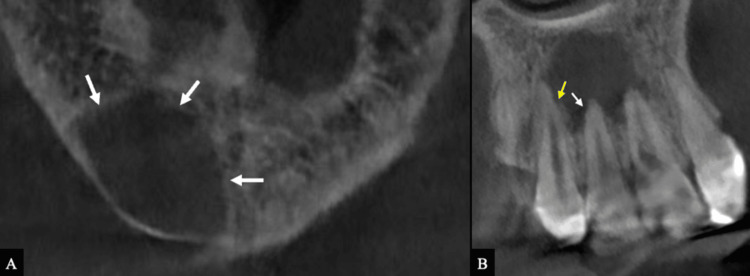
CBCT images of the anterior maxillary region. (A) Axial view demonstrating a large periapical radiolucency with thinning of the labial cortical bone (white arrows). (B) Longitudinal view demonstrating external root resorption in multiple forms, including EIR affecting teeth #11 and #12 (white arrows) and ESR in tooth #13 (yellow arrow). CBCT: Cone beam computed tomography
